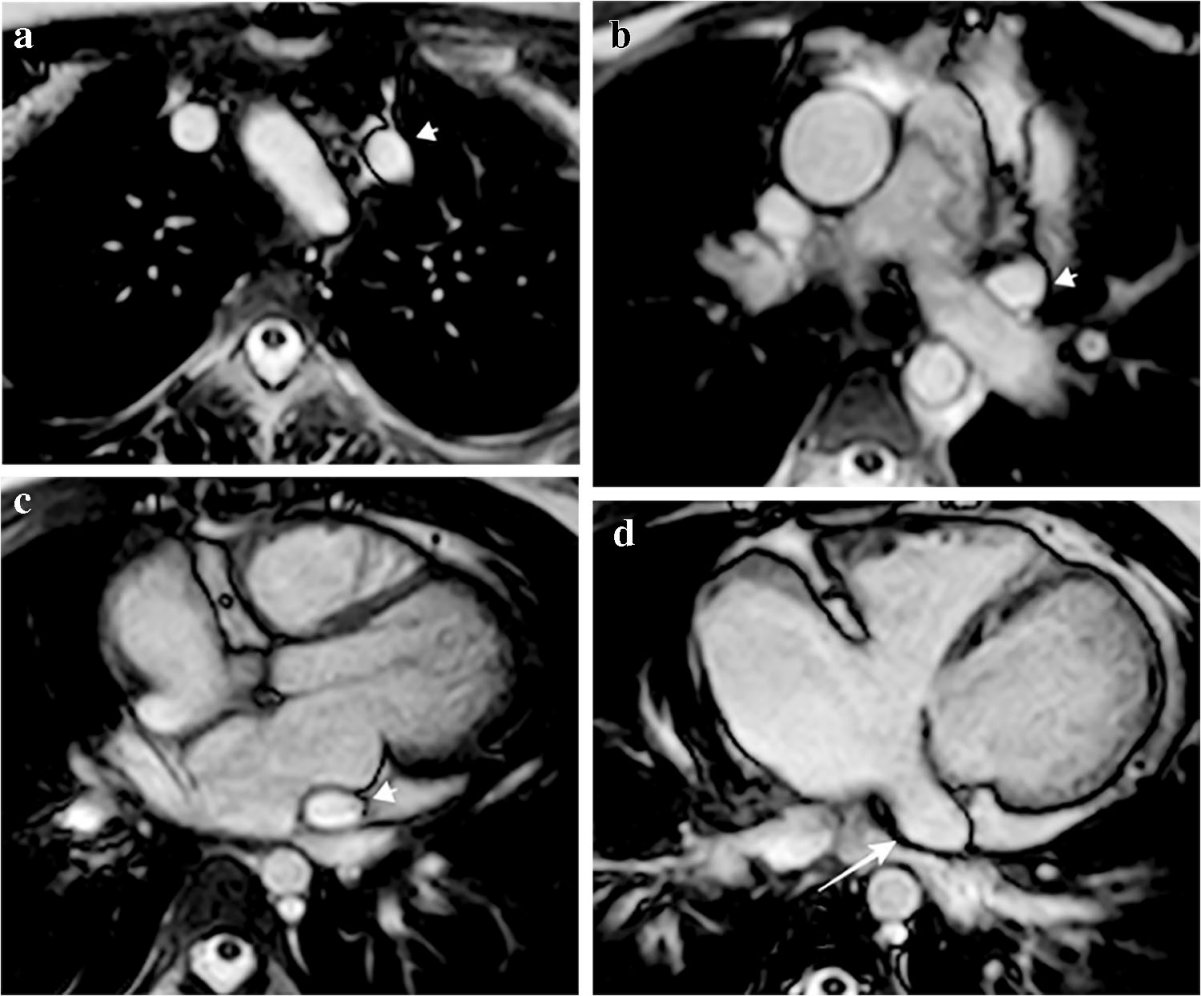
A persistent left superior vena cava (SVC) with no other anomalies in a 12-year-old girl. a–d Axial cine steady-state free precession cardiac magnetic resonance images show a persistent left SVC (*short arrow*) along its course on the left side of the mediastinum lateral to the aortic arch (a), anterior to the left hilum (b), in the left atrioventricular groove (c) and draining into the right atrium via the coronary sinus (d*, long arrow*)
